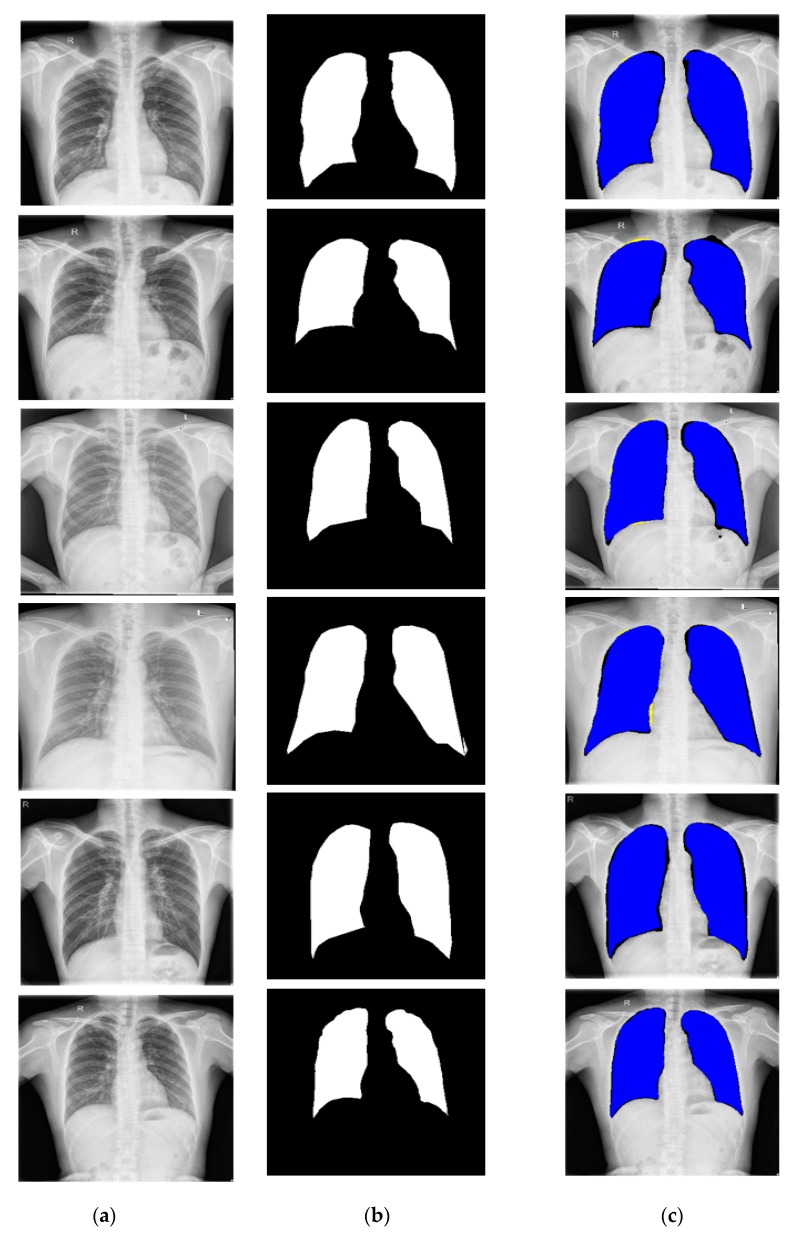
Examples of lung segmentation by X-RayNet for the SC dataset: (a) original image; (b) ground-truth mask; (c) segmented image by X-RayNet (*TP* is presented in blue, *FP* in black, and *FN* in yellow).
